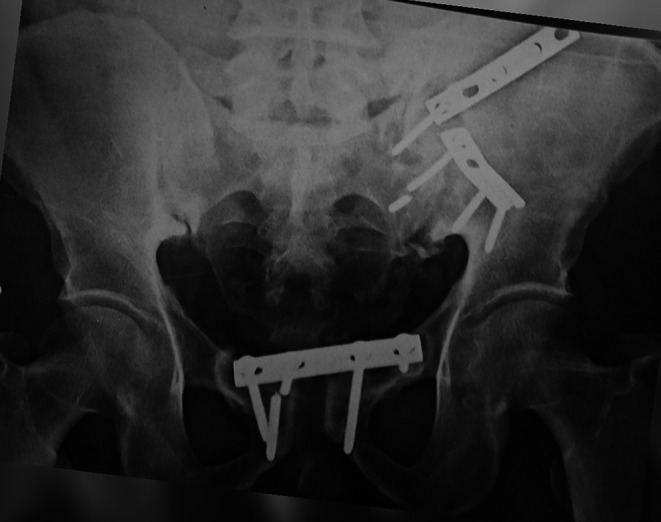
Last follow up plain radiograph showing metal failure of symphyseal plate
